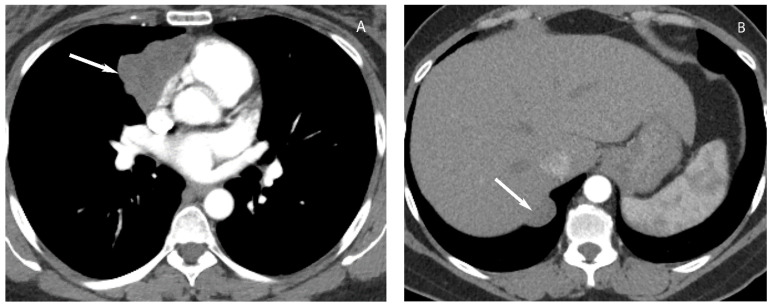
Thymoma with pleural drop metastasis. CT (A,B) shows a lobulated soft tissue mass in the prevascular mediastinum (arrow in (A)) and a diaphragmatic pleural nodule in the right lower chest (arrow in (B)).
